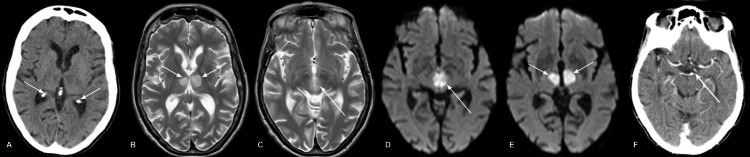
CT and MR imaging of the patient. Third CT scan showing hypoattenuation in both thalami (A). MRI-T2 showing bilateral thalamic infarcts (B) and midbrain infarcts (C). DWI-MRI showing bilateral midbrain infarcts (D and E). MR angiogram showing occlusion of the left posterior cerebral artery supplying the artery of Percheron (F). CT: computed tomography; MRI: magnetic resonance imaging; DWI: diffusion-weighted imaging
